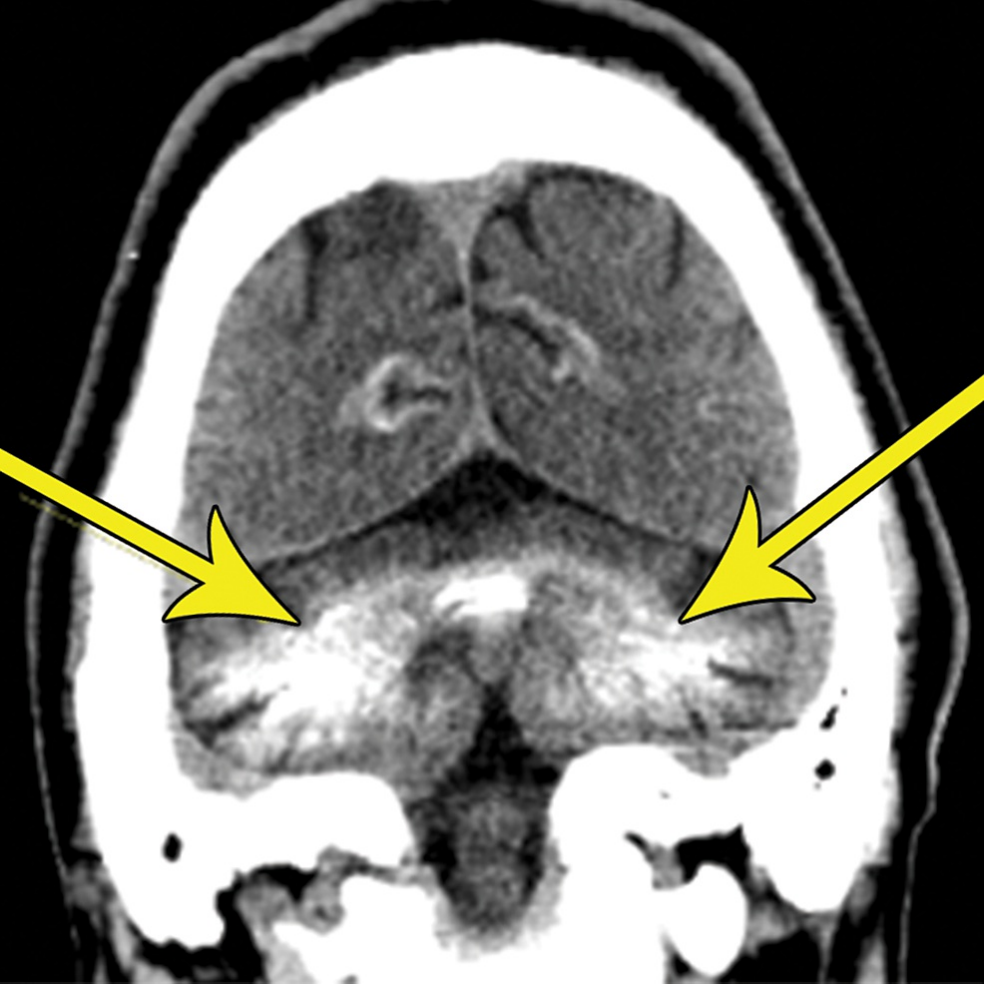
The coronal non-contrast CT scan demonstrates symmetric calcification of the cerebellum and dentate nuclei (yellow arrows) CT: computed tomography
